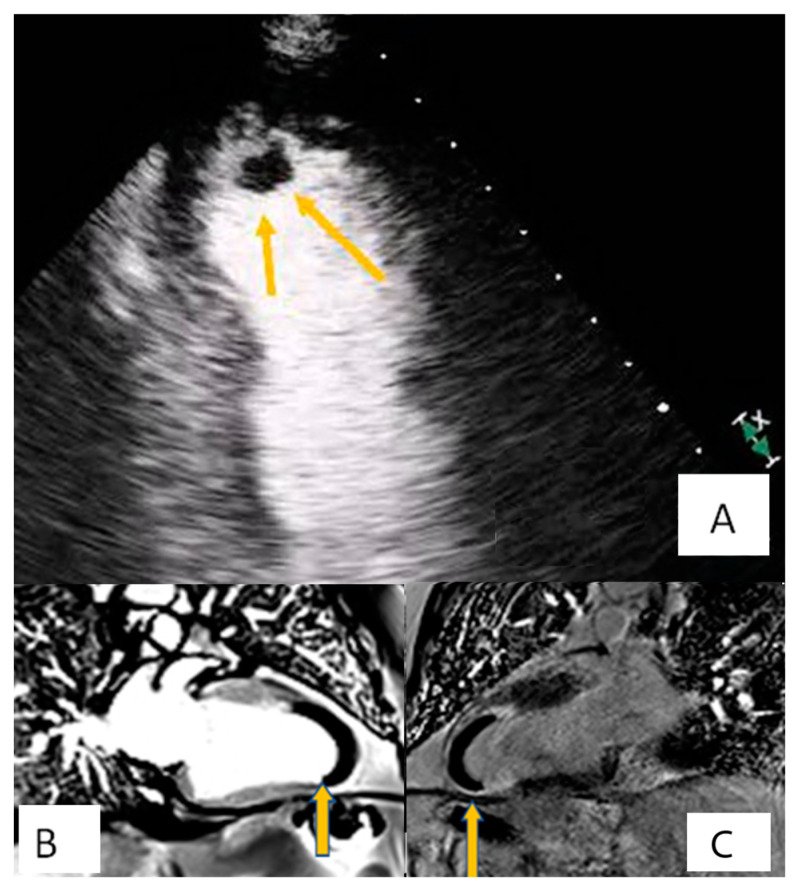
Patient presenting with anterior myocardial infarction the second day after primary percutaneous transluminar coronary angioplasty (PTCA). Apical 4-chamber view. (A) A lesion is depicted on cardiac apex (yellow arrows), mobile, without vasculature, findings compatible with clot. (B,C) Large laminar LV thrombus adjacent to an area of apical myocardial infarction. Two chambers EGE (image (B)) and LGE (image (C)) respectively.
